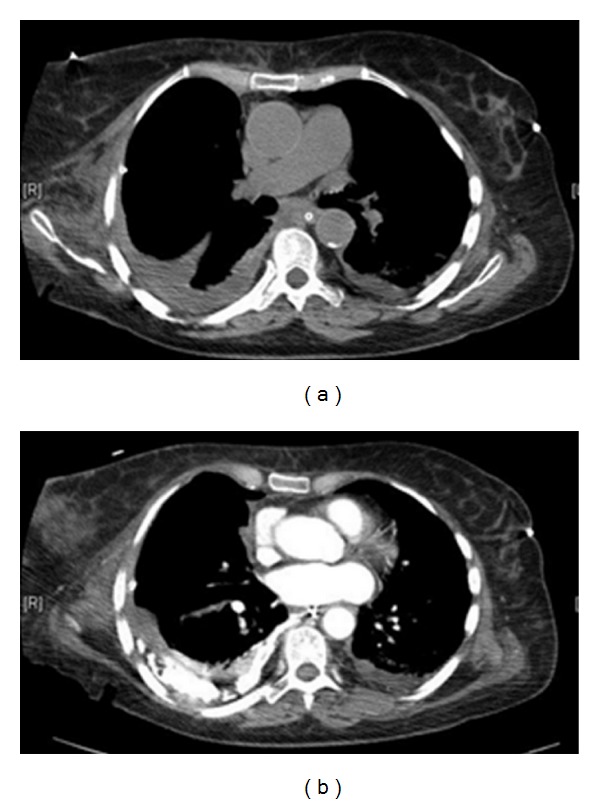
Axial CT scans of the same case as Figure 38 showing entry of water-soluble contrast into the right pleural space following esophageal perforation from an NGT.
